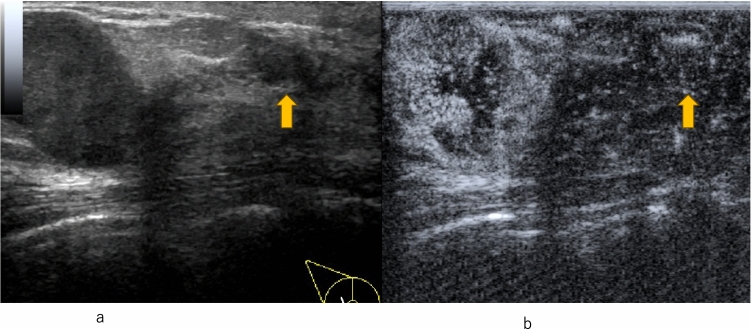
Invasive ductal carcinoma. a Conventional ultrasound shows a mass with a small hypoechoic daughter lesion (arrow). b Contrast-enhanced ultrasound of a small hypoechoic daughter lesion (arrow) shows heterogeneous enhancement
